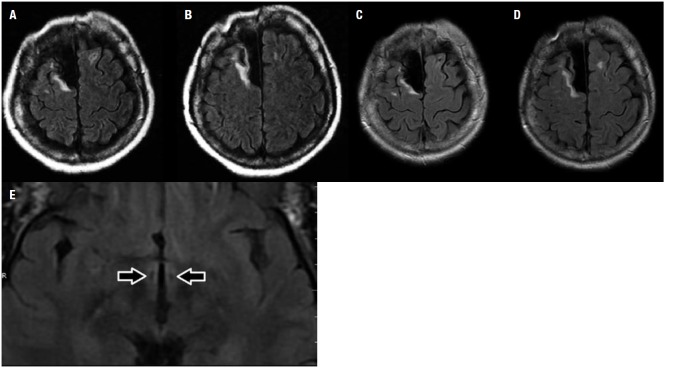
Mother's FLAIR MRI of the brain at 41 (A and B) and 49 years (C, D, and E)
